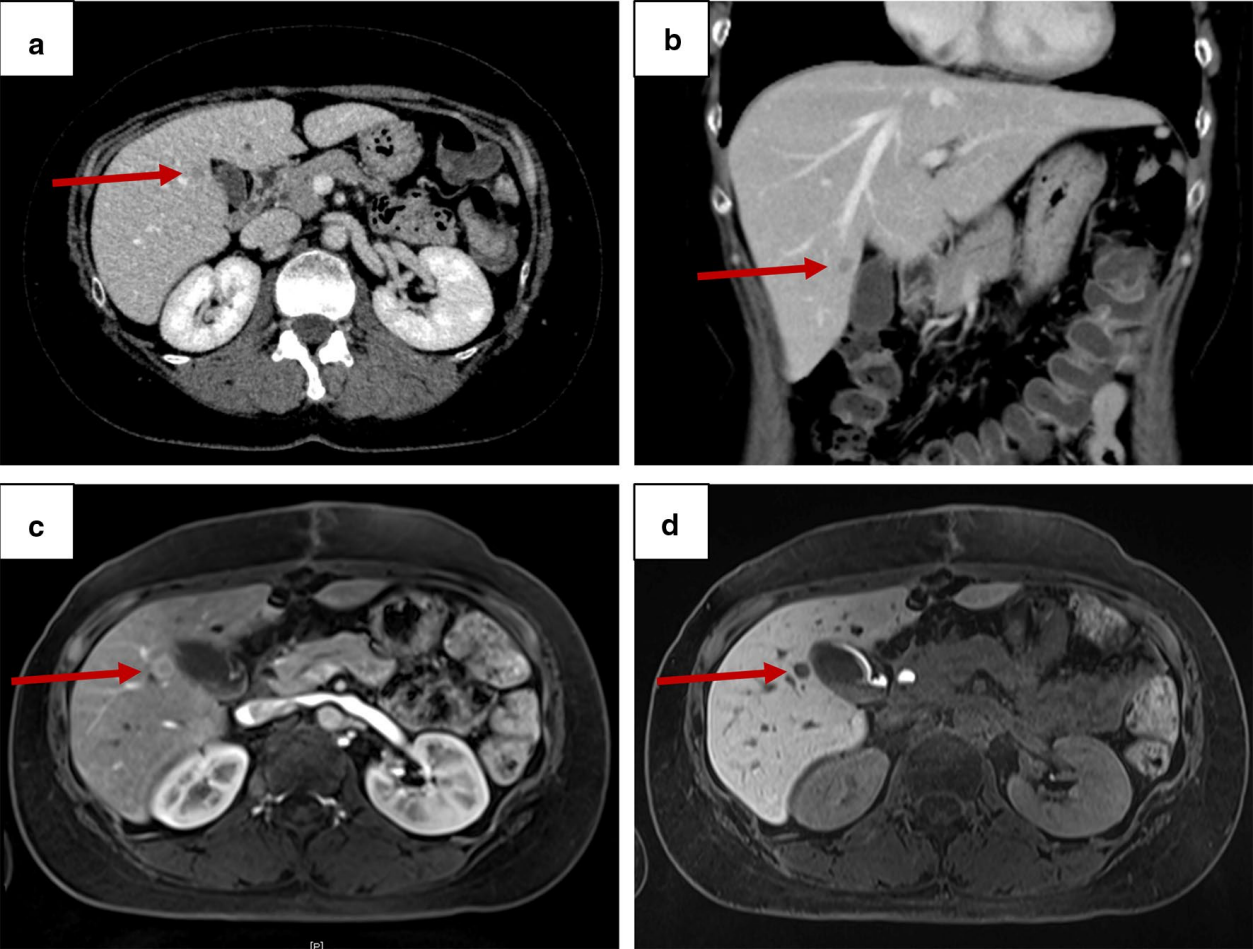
a, b Contrast-enhanced computed tomography showing a low-density area in the segment 5 of the liver. a Axial, and b coronal images. c, d Gadoxetic acid-enhanced magnetic resonance imaging showing a solitary c hyperenhancing lesion on arterial phase and d defect on hepatocyte phase. Arrows indicate the lesion
